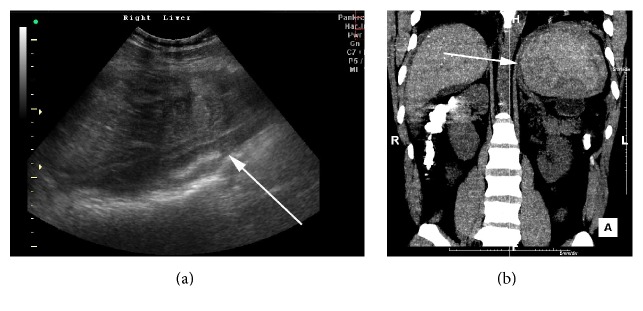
(a) USS showing a heterogenous liver and the presence of haemorrhagic fluid. (b) Computed tomography coronal reconstruction showing haemorrhage around the ruptured spleen. The arrow in (a) refers to haemorrhagic fluid in the liver and the arrow in (b) refers to the spleen.
